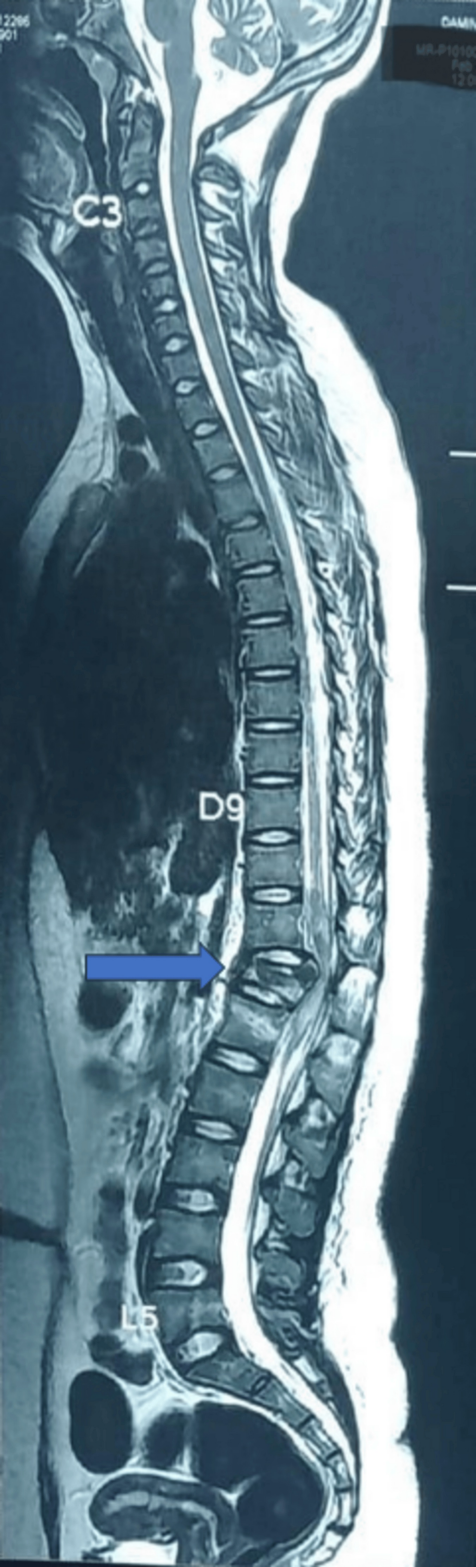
Sagittal MRI spine showing fracture at D12/L1, blue arrow (Case 3) MRI: magnetic resonance imaging; C: cervical; D: dorsal/thoracic; L: lumbar
